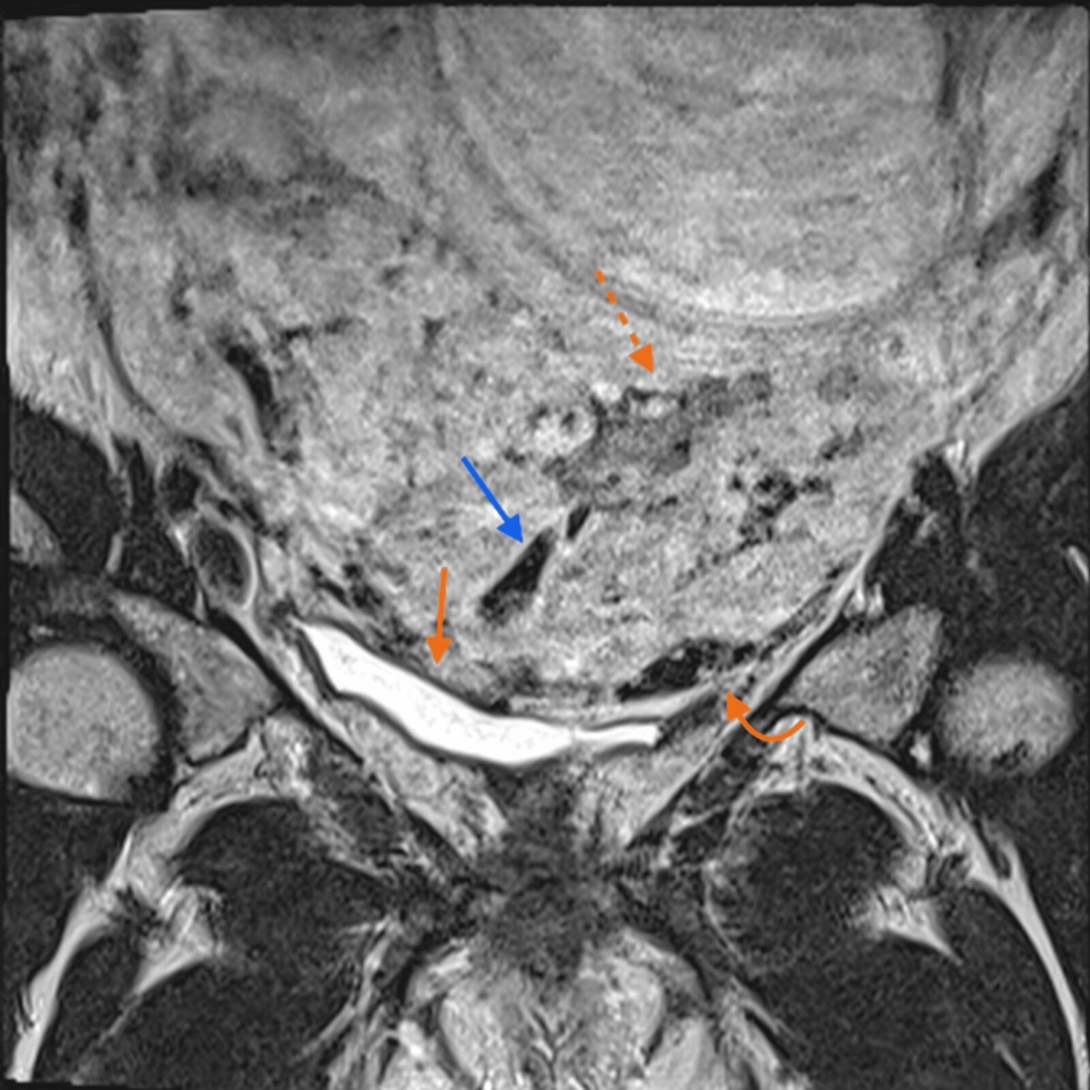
T2-weighted magnetic resonance image without fat saturation in the coronal plane. Placenta with a heterogeneous appearance due to the presence of hypointense bands (dotted orange arrow) and flow voids related to anomalous intraplacental vessels (blue arrow) as well as anomalous vessels of the placental bed (curved orange arrow). At this level, the bladder wall is intact, preserving the normal hypointense line of the bladder wall (orange arrow).
